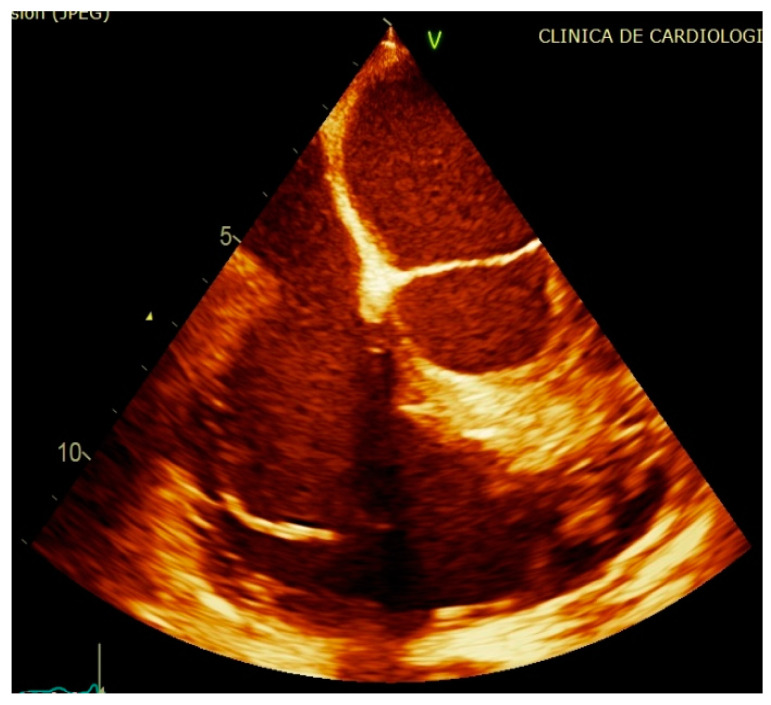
Transesophageal two-dimensional echocardiography: tricuspid chordae rupture, no residual vegetation, no pericardial effusion.
